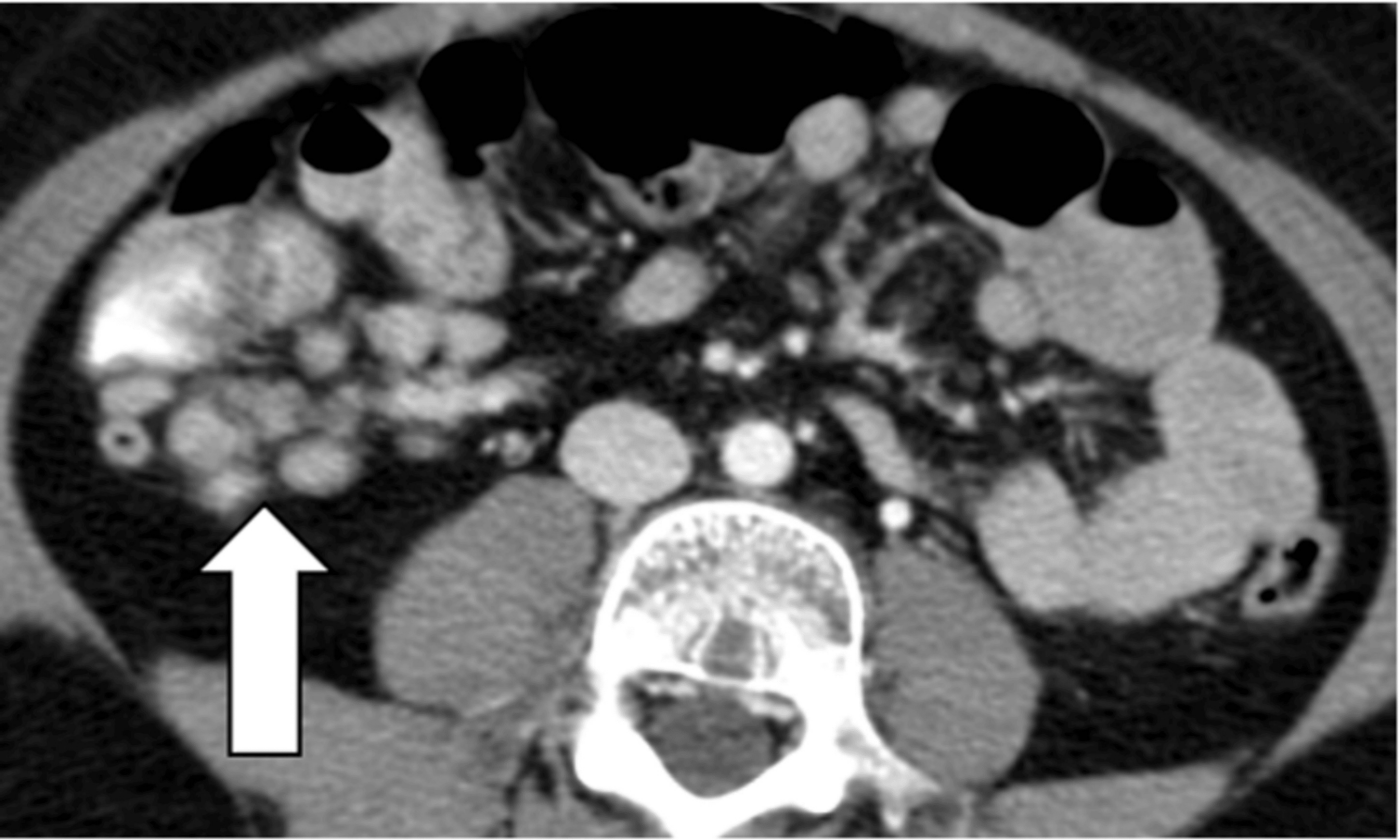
Mesenteric adenitis. CT image shows multiple ileocecal nodes greater than 5 mm in diameter (white arrow), adjacent to a normal, air-filled appendix.
